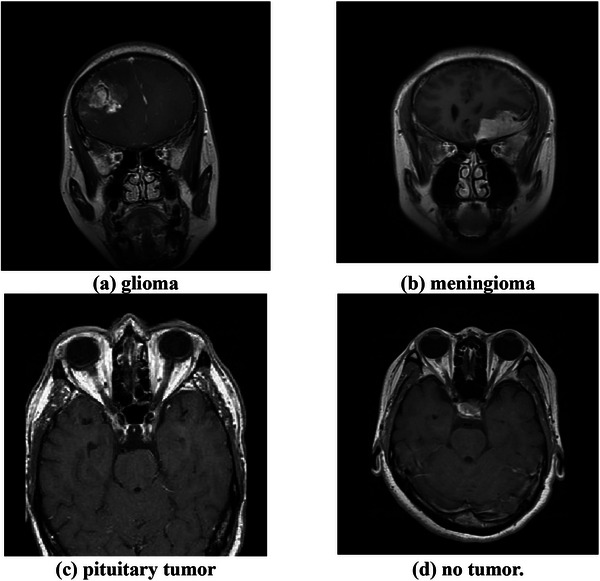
Representative T1‐weighted MRI scans from the Kaggle Brain tumor MRI dataset: (a) glioma, (b) meningioma, (c) pituitary tumor, (d) no tumor.
